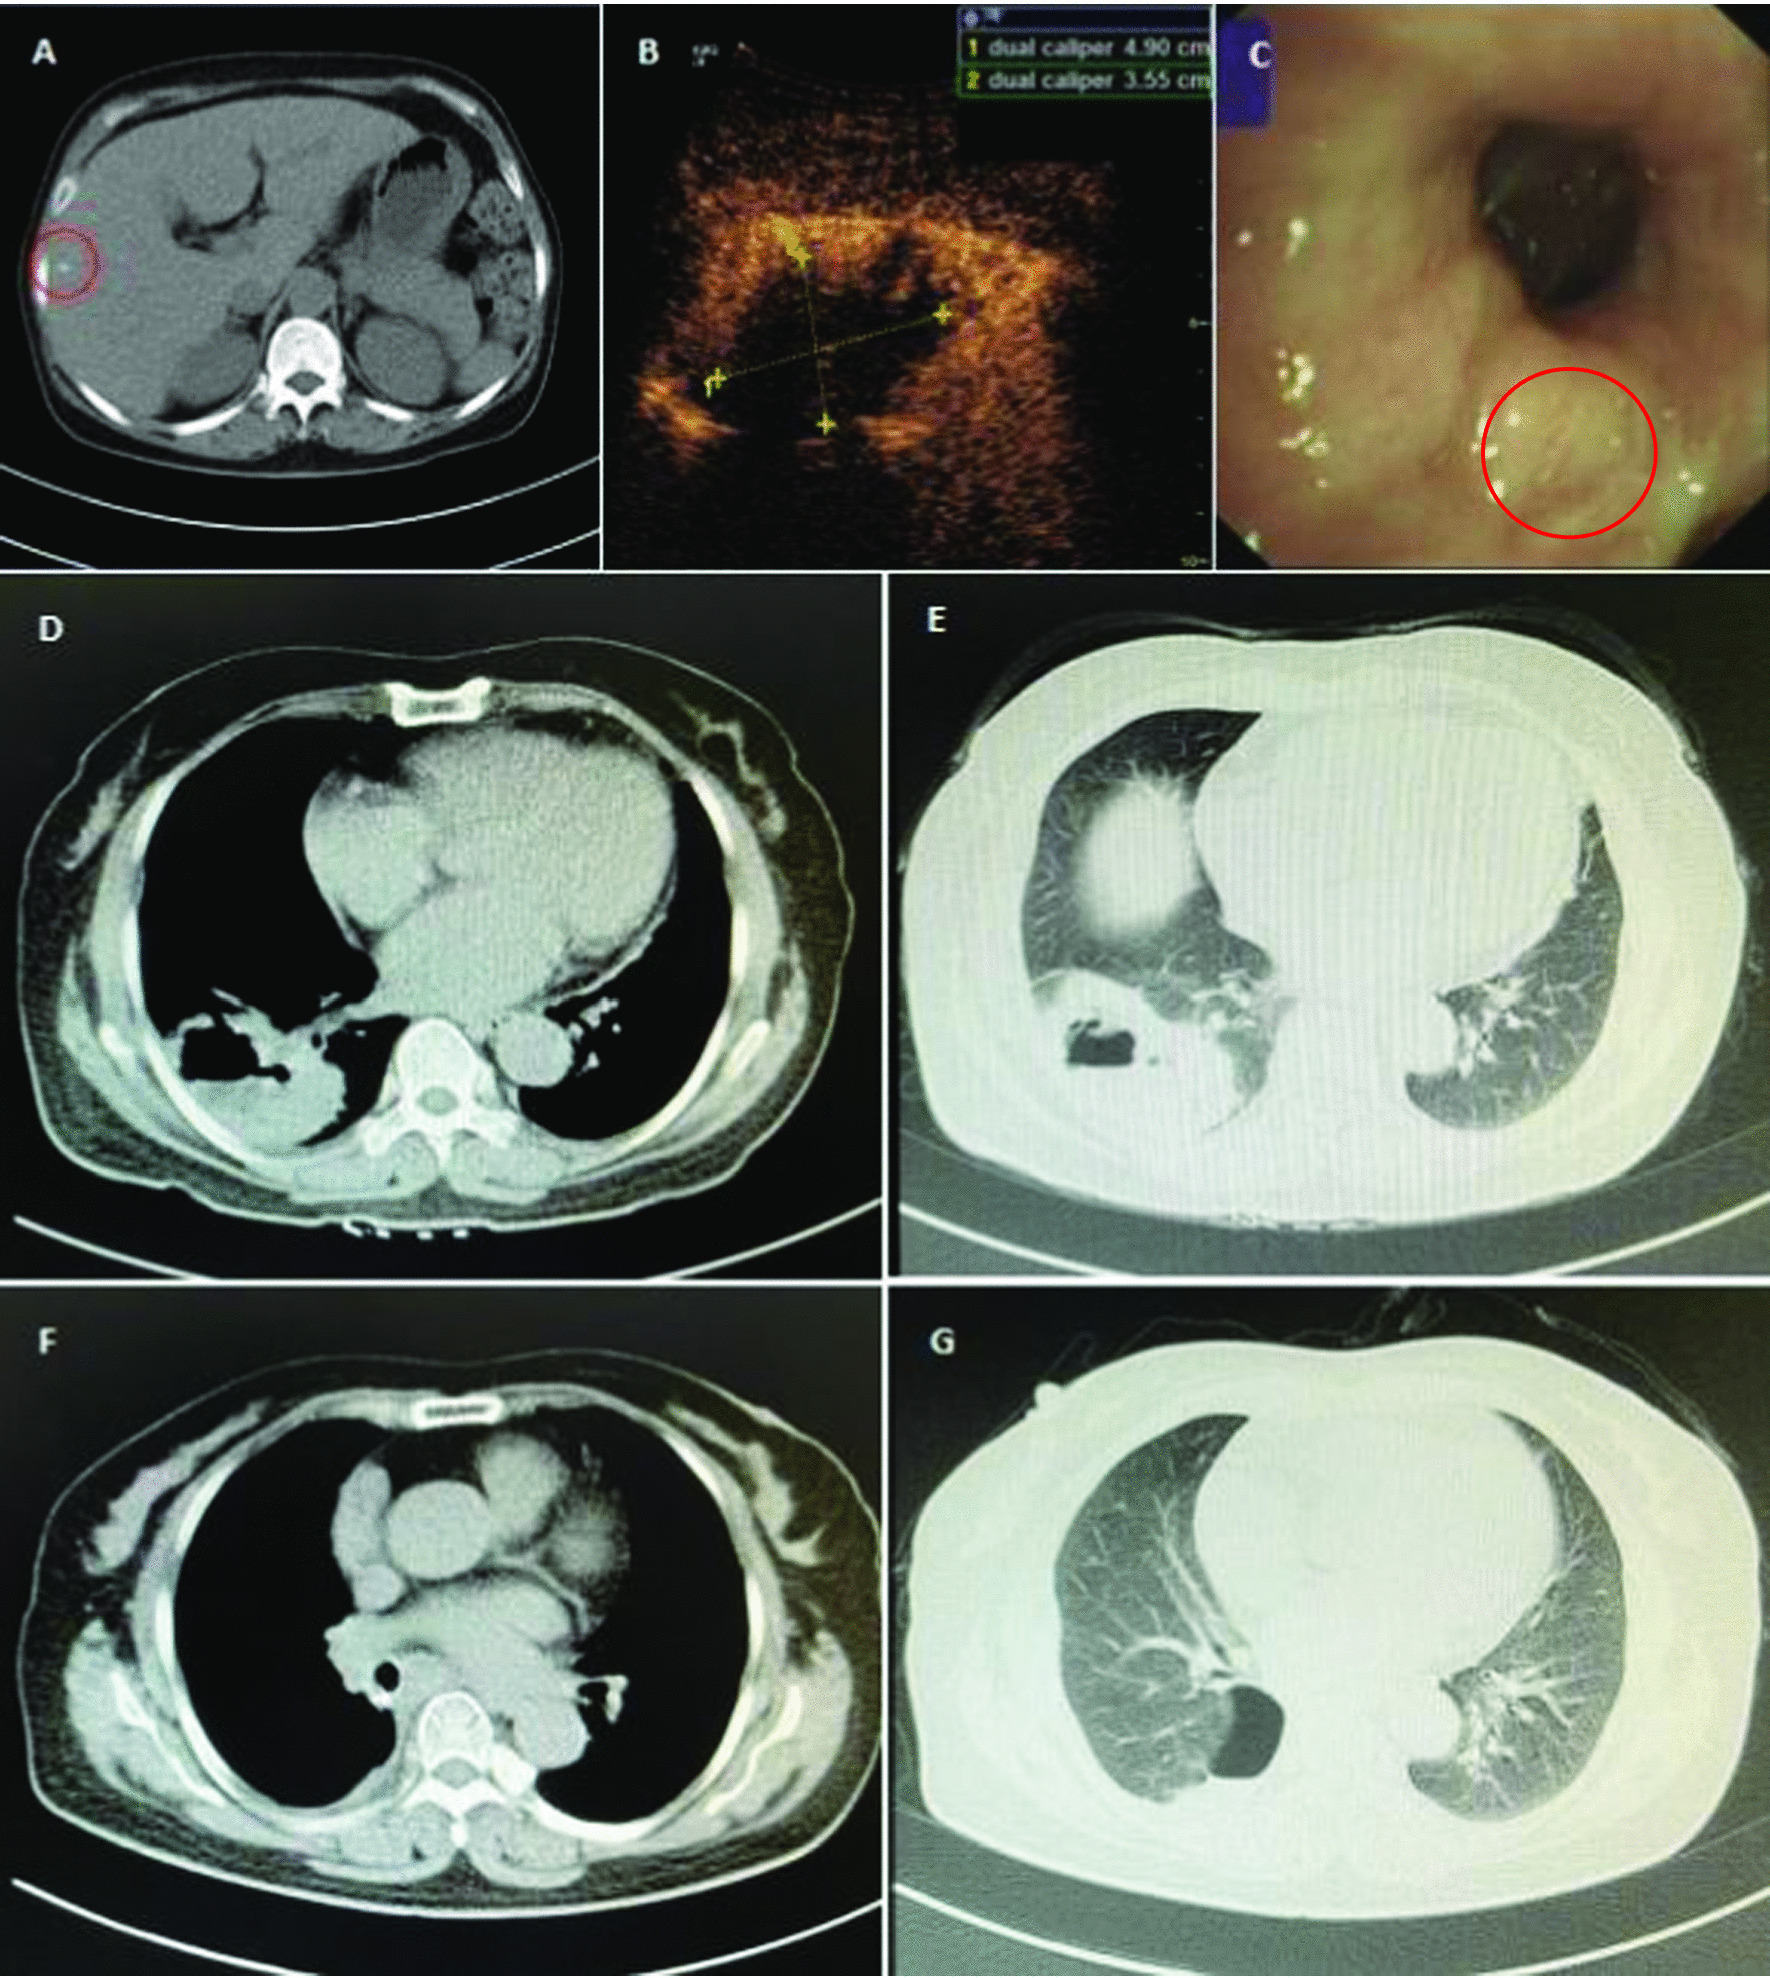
CT scan images. Abdominal CT images taken before surgery, and the circled area showed a small nodule (A). Chest color Doppler ultrasound images, the size of the pulmonary lesion was 4.9 cm × 3.55 cm, and necrotic tissue was shown in the center of the lesion (B). Bronchoscopy image of the lower right lobe of bronchial lumen, the circled area showed white mucus (C). Chest CT images taken before surgery (D, E). Chest CT images taken on follow up visit in September (F, G)
